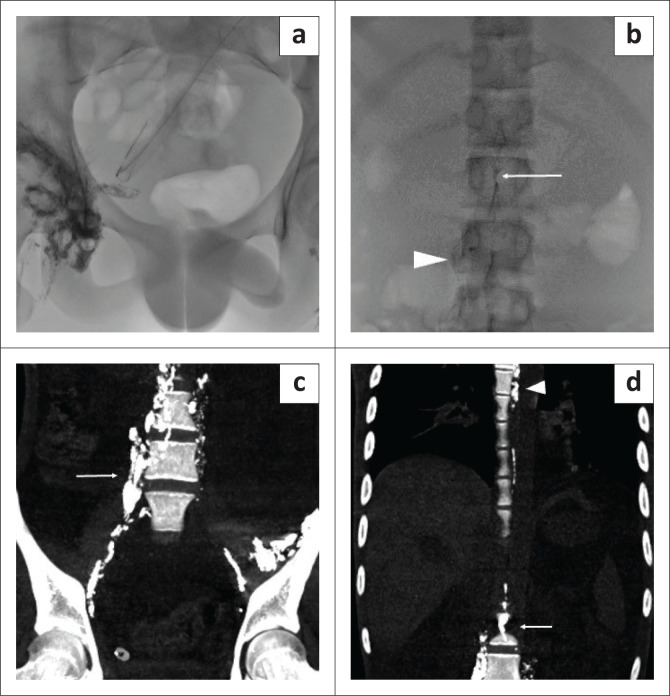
Fluoroscopic nodal lymphangiography was performed in the same child for diagnostic and therapeutic purposes. (a) Fluoroscopic images showed contrast injected in bilateral groin nodes. (b) Fluoroscopic image 30 min after intranodal lipiodol injection depicted a normal cisterna chyli (arrow) and lumbar lymphatics (arrowhead). No intraperitoneal contrast leak was seen. Coronal reformatted CT images post fluoroscopic lymphangiography with thin MIP reconstruction of the abdomen and pelvis (c) and chest (d) demonstrated normal opacification of the lumbar lymphatics (arrow in c) and normal opacification of the cisterna chyli (arrow in d) and thoracic duct (arrowhead in d).
